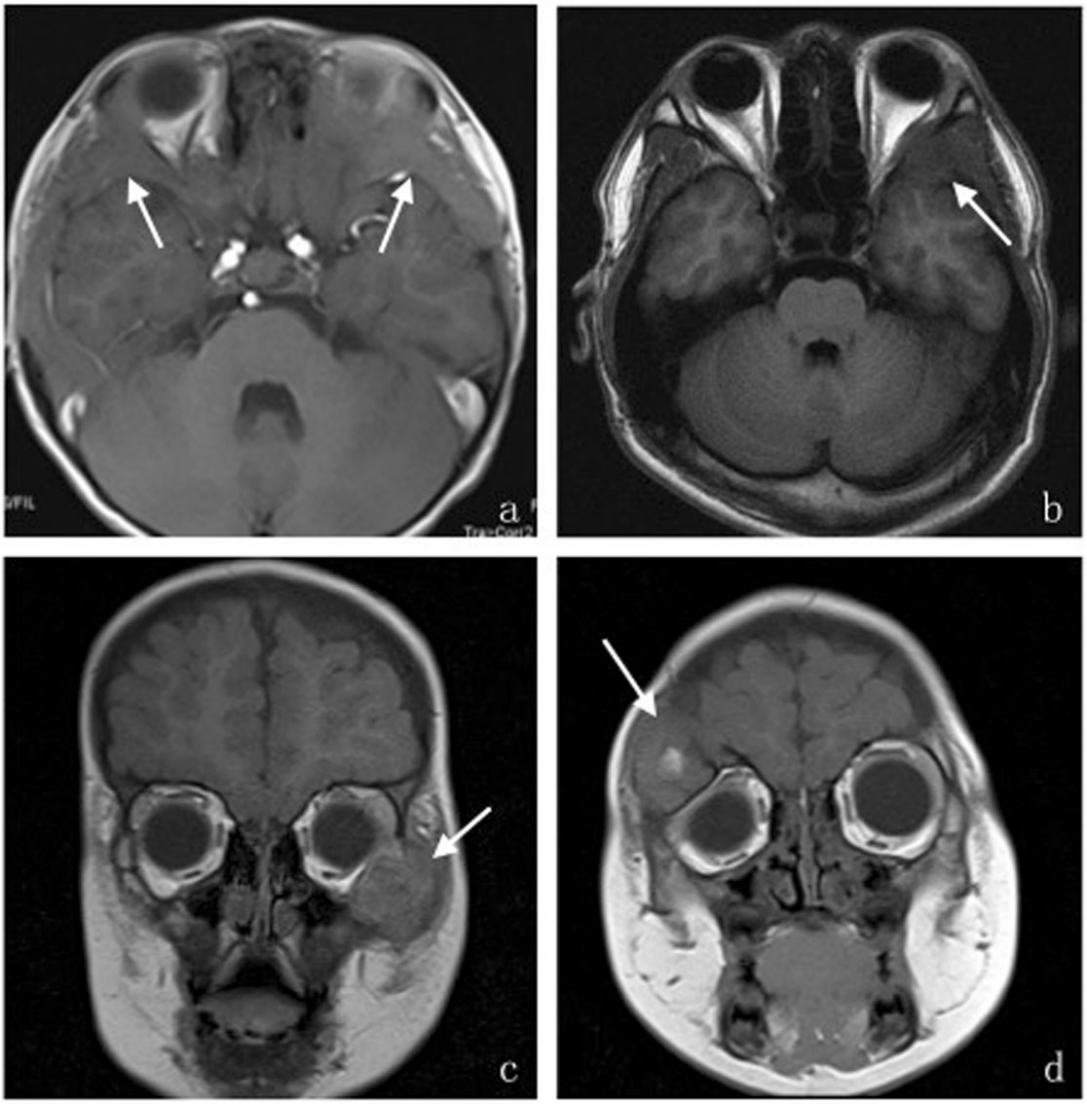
a–d. The different performances in T1WI.Axial (a) T1-weighted MR images show isointensity, and the lesion performs diffuse thickening of the affected bone with ill-defined margins; Axial (b) T1WI show lesion located in the left lateral orbital wall presenting hypointensity and an unclear boundary; Coronal (c) T1-weighted MR images show mass located in the inferolateral left orbit presenting iso-hypointense mixed signals and an unclear boundary. Coronal (d) T1WI show lesion located in the superolateral right orbit performing isointensity with patchy hyperintensity inside
